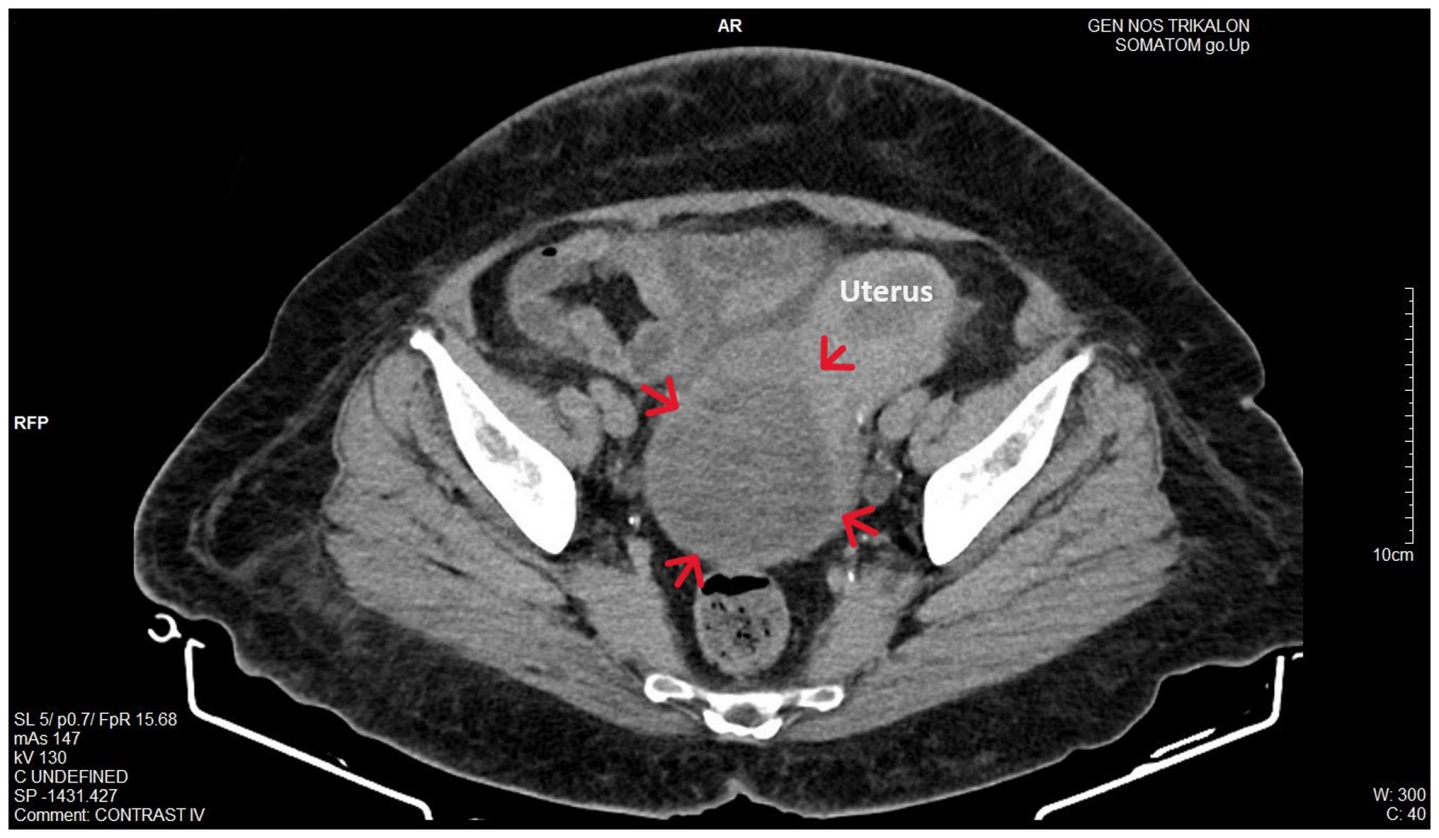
Computed tomography imaging of a large prolapsed pedunculated submucosal uterine leiomyoma: Significant distention and non-homogeneity of the cervix (red arrows) with thinning of the external wall is depicted.
